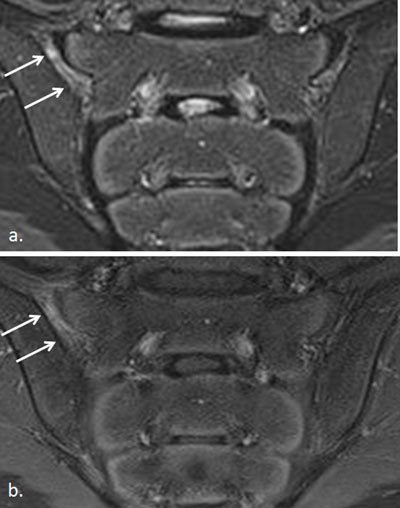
Active sacroiliitis with synovitis/retroarticular enthesitis in a 14-year-old boy with JSpA according to a global assessment of MRI for sacroiliitis, not according to the ASAS definition of a positive MRI for sacroiliitis. a. Semicoronal STIR MR image demonstrates synovitis/retroarticular enthesitis of the right sacroiliac joint (arrows). b. Semicoronal contrast-enhanced fat-saturated T1-weighted image shows synovial enhancement and enhancement of the retroarticular interosseous ligaments (arrows).
